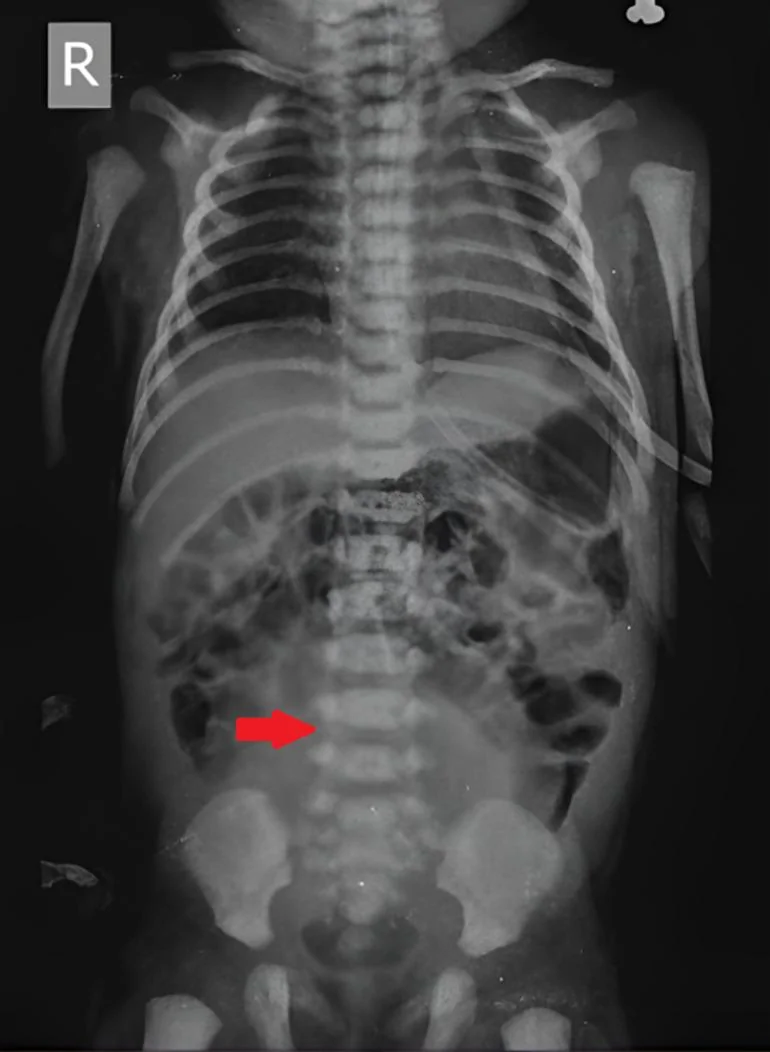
Plain anteroposterior chest X-ray demonstrates a pelvic opacity with mass effect on adjacent intestinal loops
